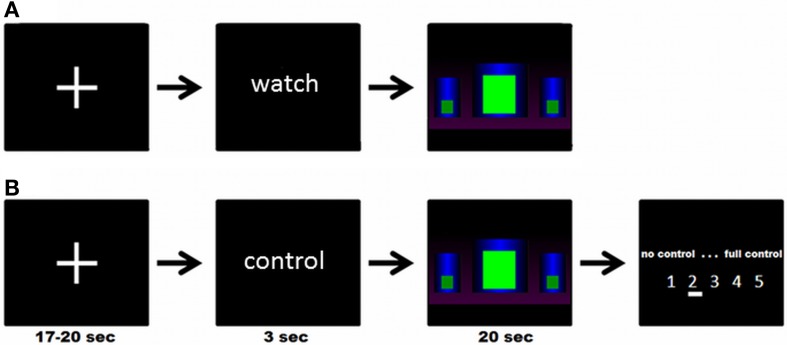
(A) Example of a complete trial of the “watch moving/static bars” condition; (B) Example of a complete trial of the “get control” condition; participants were instructed to try to control the movements of the bars during functional MRI measurement.
